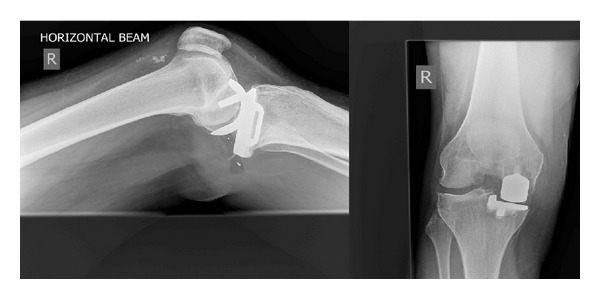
Lateral view and AP view radiographs of the knee taken upon presentation at the Accident and Emergency Department.
